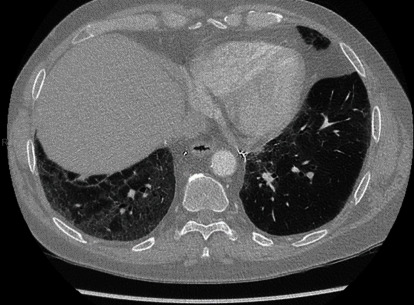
CT chest imaging 2 years (12/09/2018) posttransplant.
